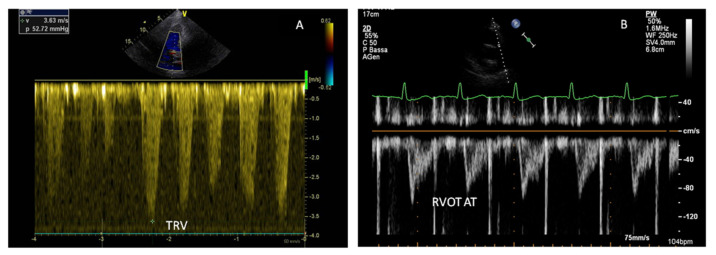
Echocardiographic markers of pulmonary hypertension (PH). (A) Increased peak tricuspid regurgitation velocity (TRV); (B) reduced right ventricular outflow tract (RVOT) acceleration time.
